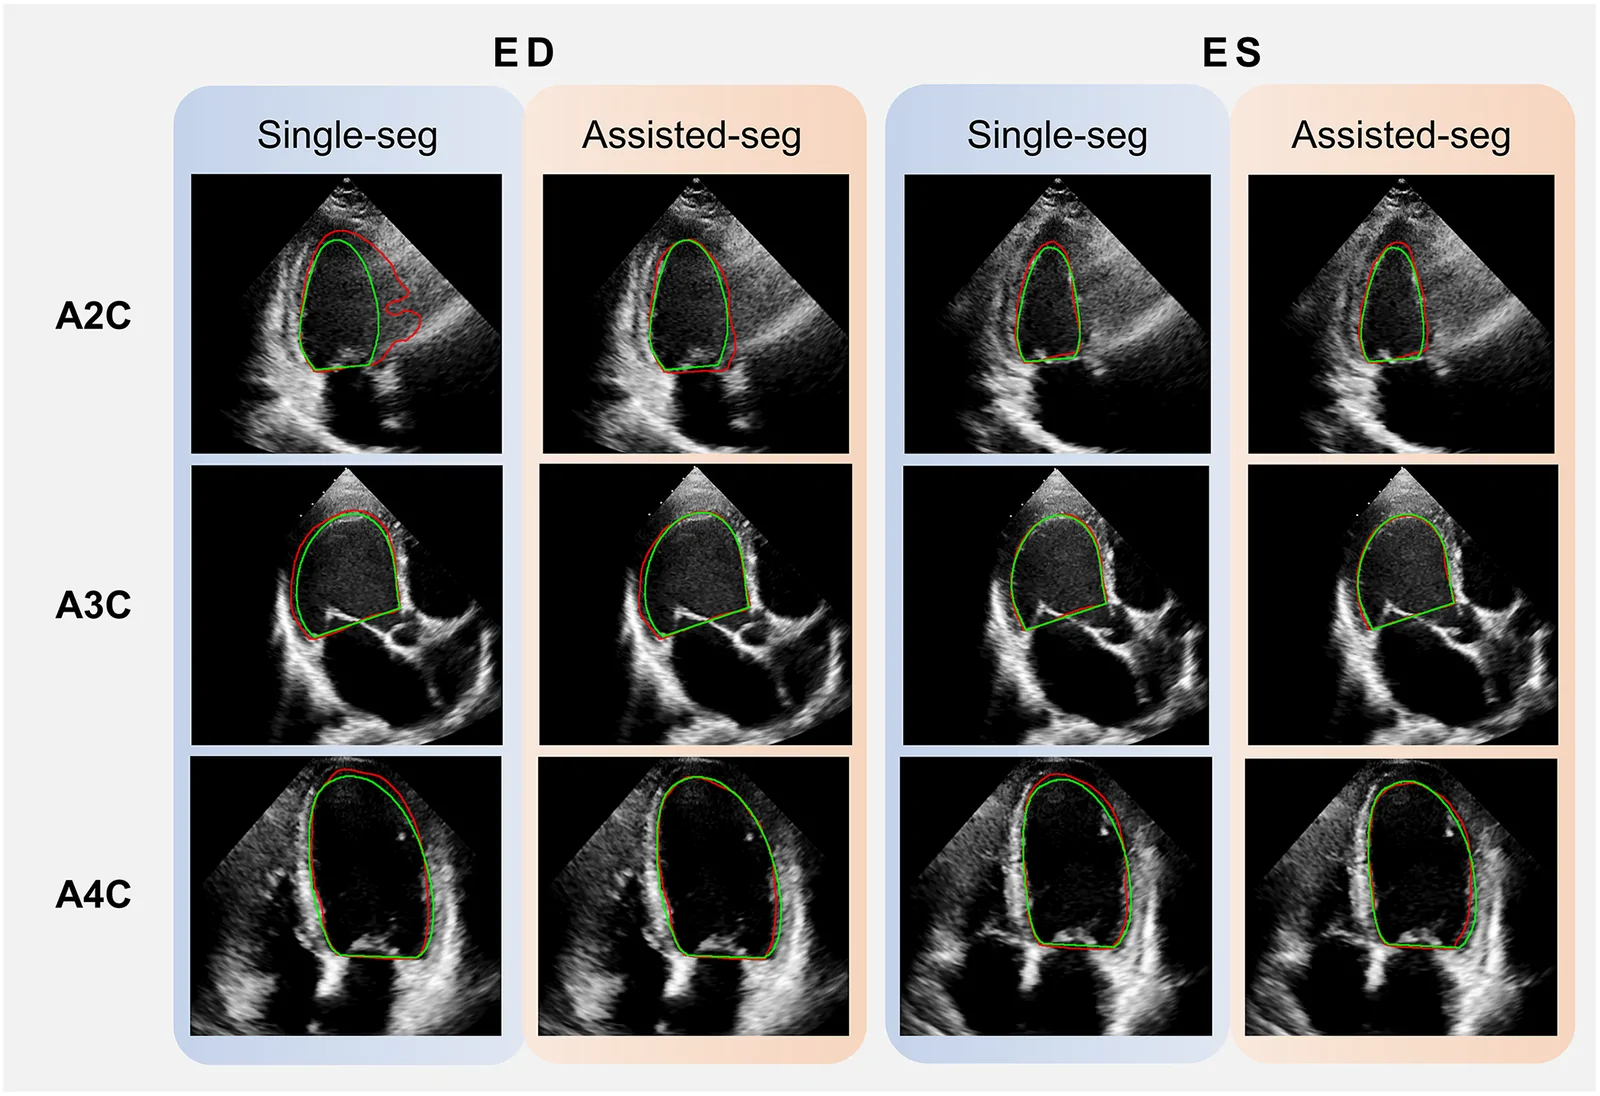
Visualization of the improvement in segmentation with the optical flow module in the apical 2-chamber, 3-chamber, and 4-chamber views. Single-seg, segmentation without optical flow network assistance; Assisted-seg, segmentation with optical flow network assistance; ED, end-diastole; ES, end-systole. Predicted results are shown in red, and manual annotations in green.
